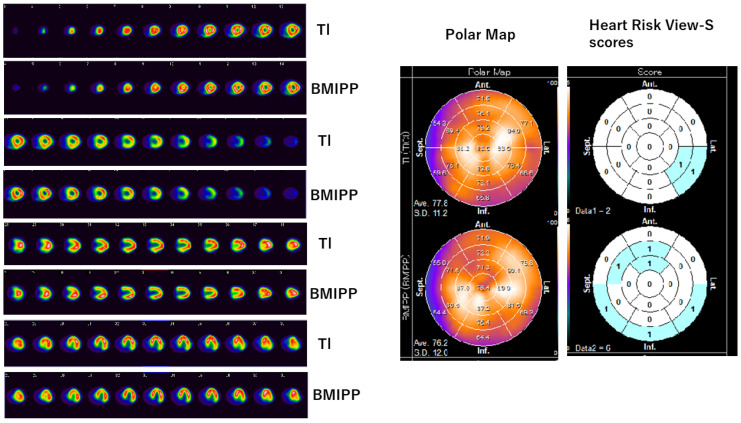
Tl/BMIPP dual-isotope myocardial scintigraphy of another patient. (Left) SPECT images are arranged in order from top to bottom: left ventricular short-axis planes of ^201^Tl and ^123^I-BMIPP, long-axis planes of ^201^Tl and ^123^I-BMIPP, and horizontal planes of ^201^Tl and ^123^I-BMIPP. (Middle) Polar map of ^201^Tl (top) and ^123^I-BMIPP (bottom). (Right) Heart Risk View-S scores of ^201^Tl (top) and ^123^I-BMIPP (bottom). Patient H in Table 2. Original image data can be found in Supplementary Materials (Figures S7 and S8).
